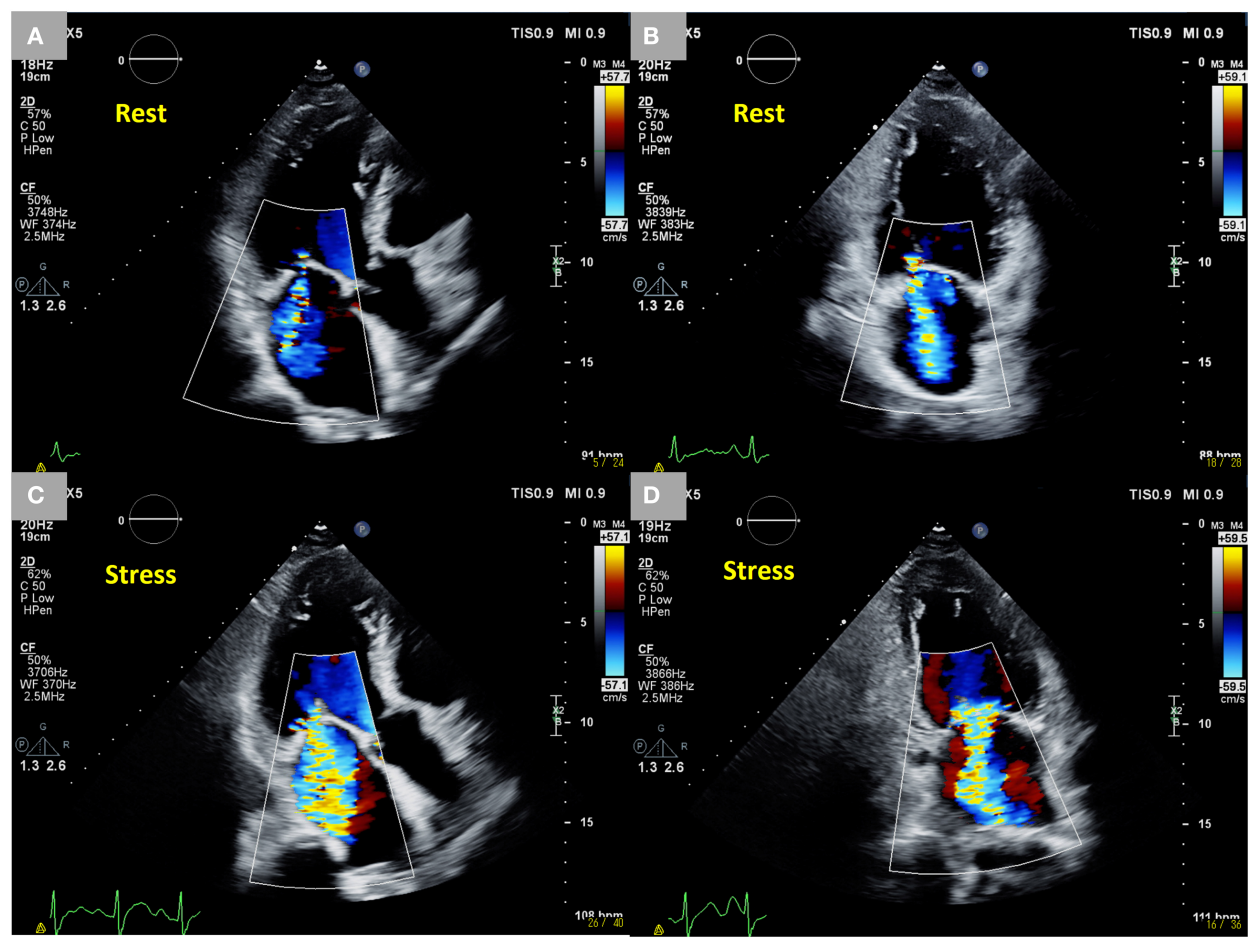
Dynamic changes in SMR during exercise stress echocardiography in an 85-year-old male patient who had an anterior old myocardial infarction and heart failure with reduced ejection fraction. Moderate SMR (EROA 0.22 cm^2^) at rest. (A,B) Two-dimensional color Doppler images from three- and two-chamber views. Dynamic severe SMR (EROA 0.46 cm^2^) under stress. (C,D) Two-dimensional color Doppler images from three-chamber and two-chamber views. SMR, secondary mitral regurgitation; EROA, effective regurgitant orifice area.
